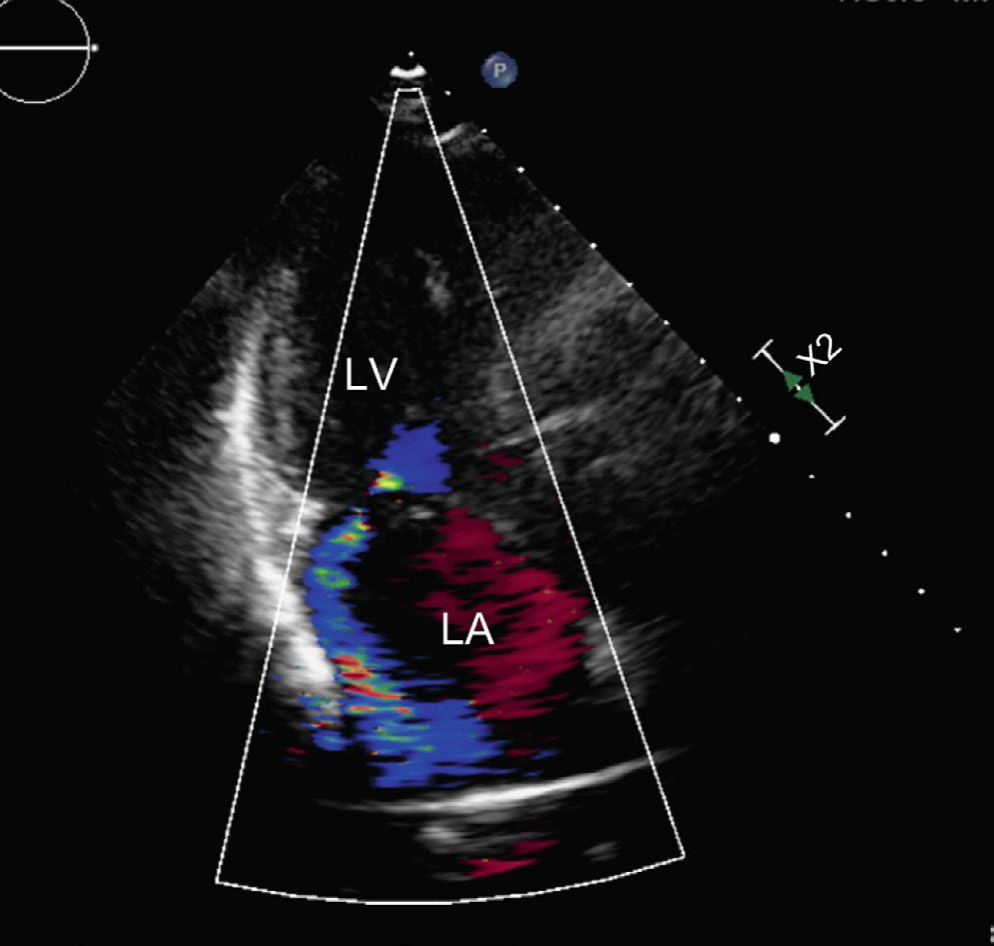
Transthoracic echocardiography findings 3 months before admission. There is moderate mitral valve regurgitation and no vegetation on any valves
